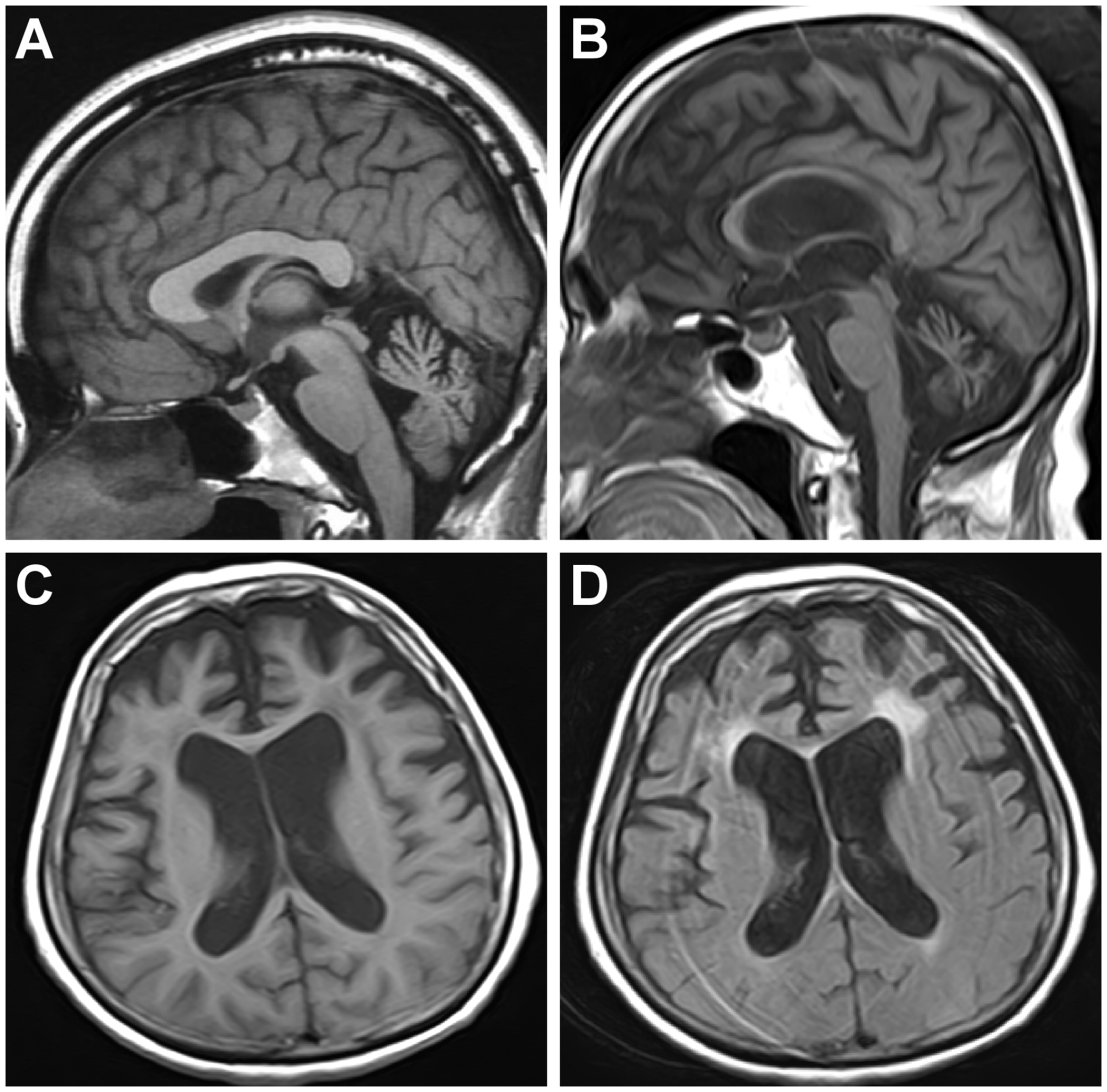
Brain MRI of the proband (IV-1) and her mother (III-3). T1WI sagittal brain MRI image showings cerebellar atrophy in the proband (IV-1, 39 years old) and her mother (III-3, 65 years old) (A, B). Additionally, T1WI and FLAIR axial images show frontal atrophy with white matter lesions in the mother (III-3) (C, D).
